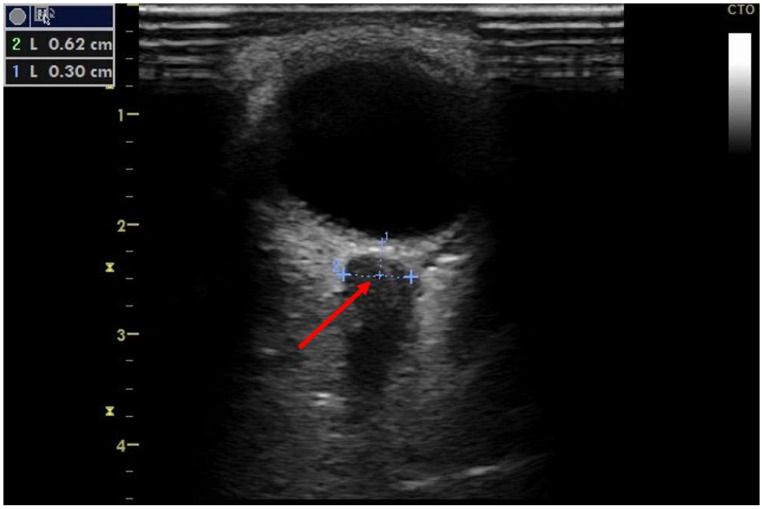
Ocular ultrasound in patient #1. Ocular ultrasound image. Optic nerve sheath diameter is measured 3 mm behind the globe at 6.2 mm (red arrow), indicating intracranial pressure above 20 mmHg.
